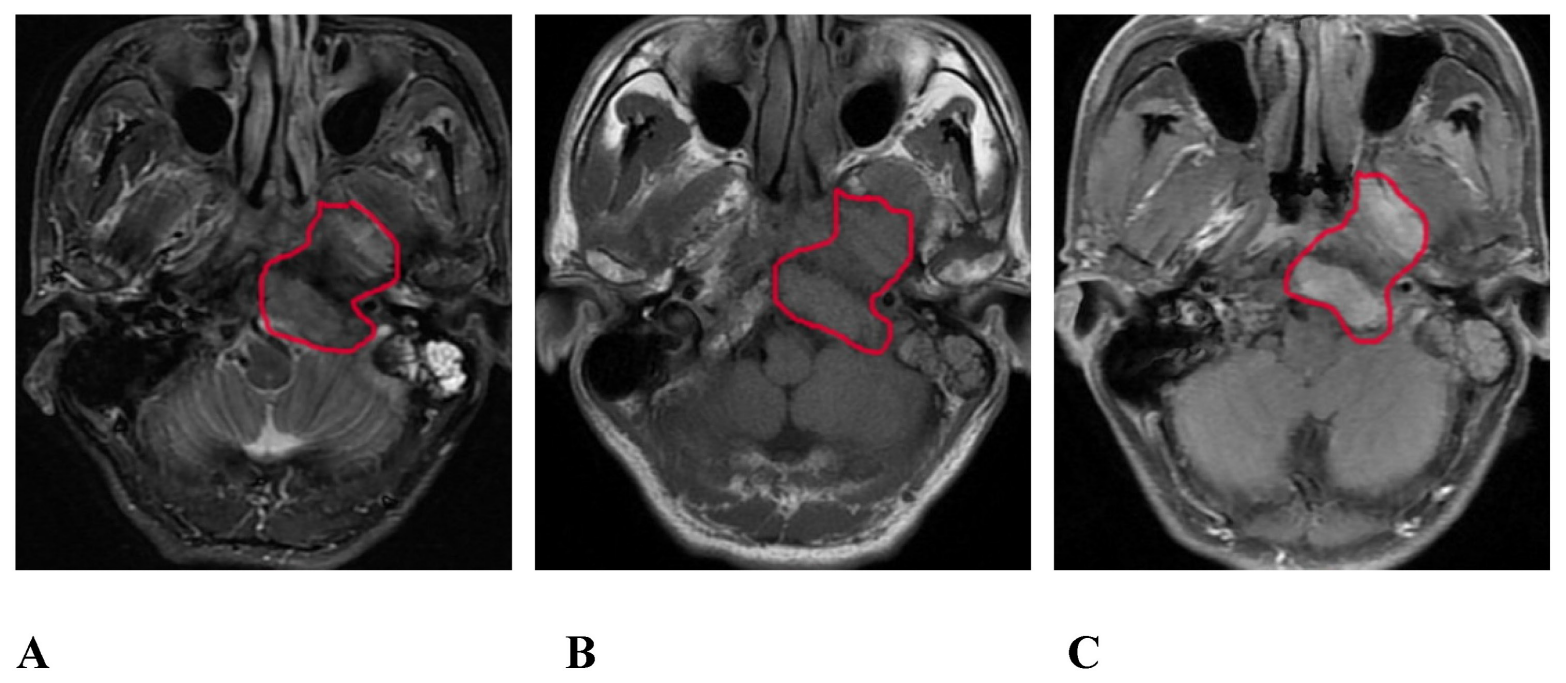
A 57-year-old male with SBI in NPC. A, B, and C show the conventional MRI sequence transverse axial STIR, T1WI, and T1WI-enhanced scan sequences, respectively. The red circle shows the clivus, the left processus pterygoidei, and the parapharyngeal muscle involvement. STIR is slightly hyperintense, T1WI is isointense, and the enhanced scan is significantly enhanced. SBI, skull-base invasion; NPC, nasopharyngeal carcinoma; STIR, short-tau inversion recovery; T1WI, T1-weighted imaging.
